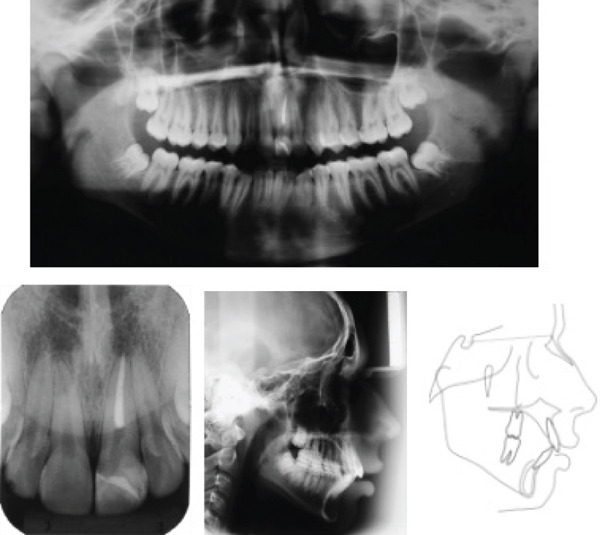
Posttreatment panoramic, periapical, and lateral cephalometric radiographs and tracing.
